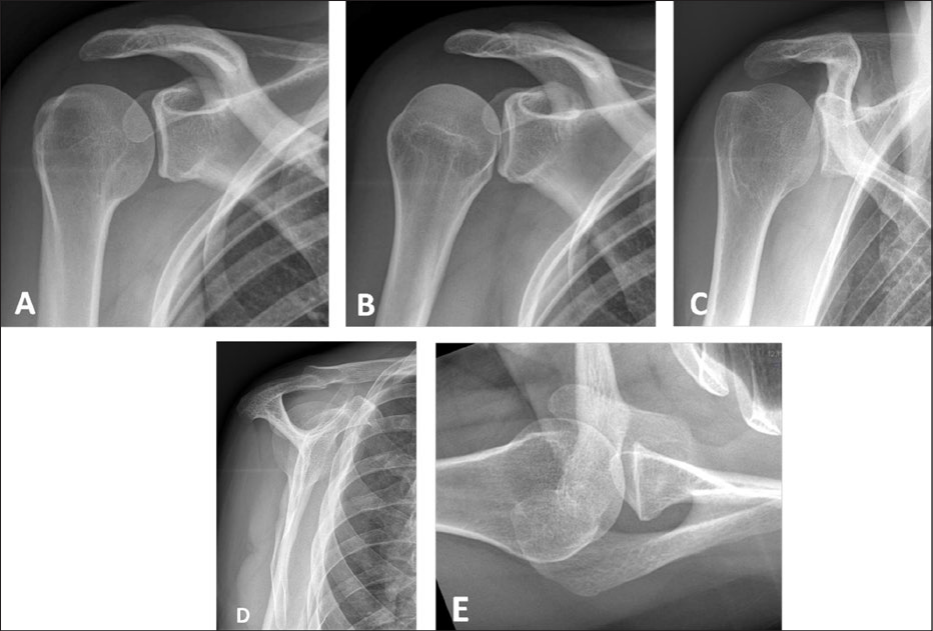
Conventional radiographs of the shoulder. (A) Anteroposterior (AP) view with external rotation; (B) AP with internal rotation; (C) AP with neutral arm position; (D) Lateral view of scapula or ‘Y’ view; (E) Axillary view.
